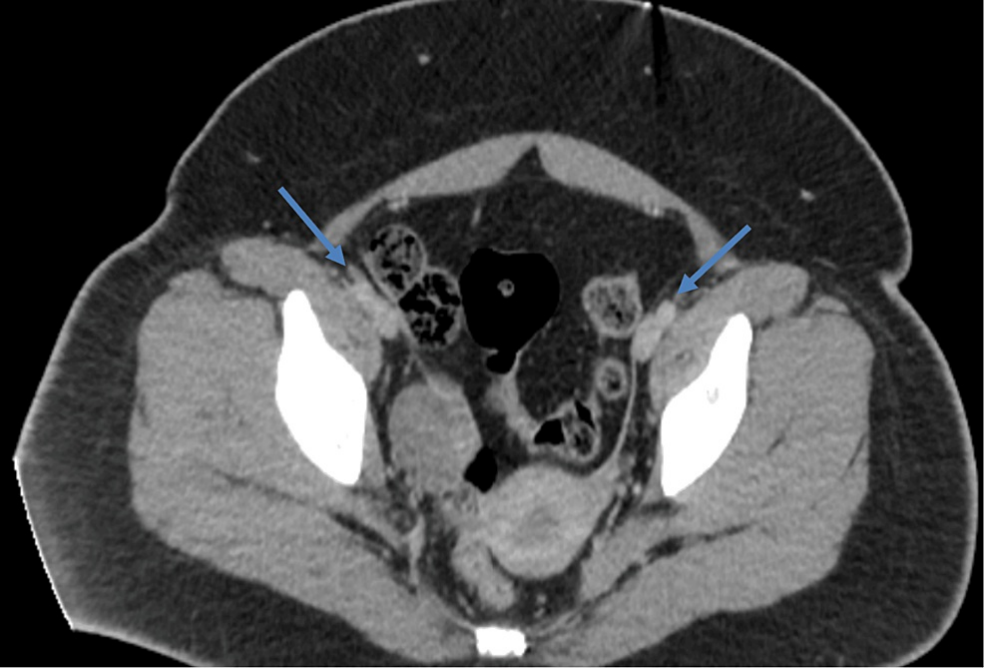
CT of the pelvis negative for pathology Arrows point to ovaries bilaterally. No mass or cyst was noted. CT: computed tomography
